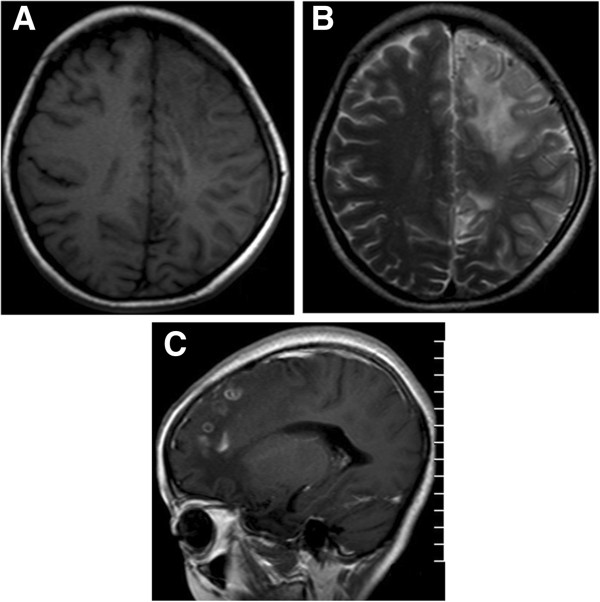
MR images for the case 4. A. The T1W image shows there is an irregular large patchy area with hypointensit in the left frontal lobe including the areas of the white matter, gray-white matter junction, and gray mater. B. The T2W image shows there is an irregular large patchy area with hyperintensity in the left frontal lobe including the areas of the white matter, gray-white matter junction, and gray mater. C. The enhanced T1W image shows a few ring-enhanced lesion areas.
